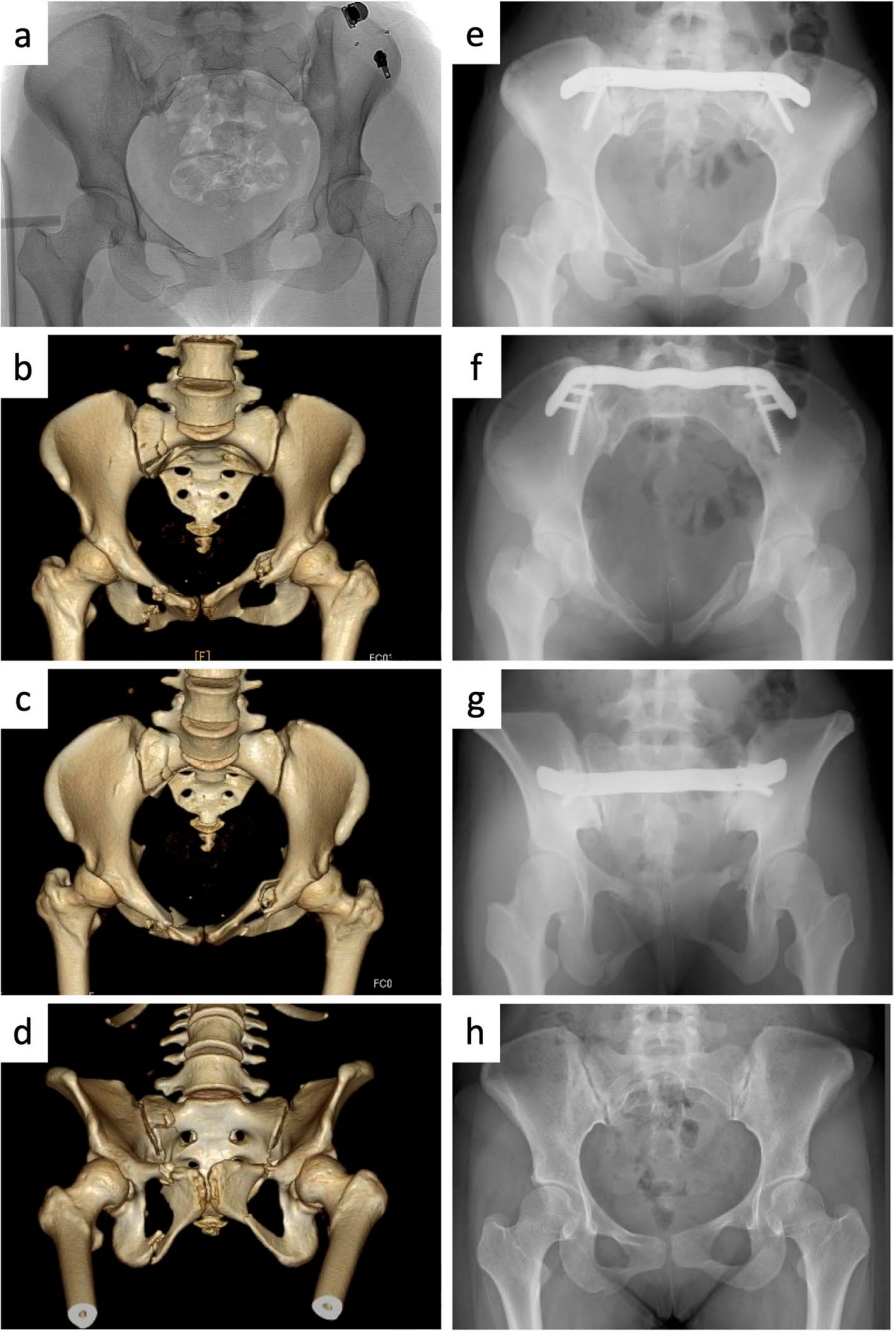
Case example. a Plain AP radiograph at the emergency room. b 3D reconstruction of the CT scan (AP view). c 3D reconstruction of the CT scan (inlet view). d 3D reconstruction of the CT scan (outlet view). e Postoperative radiograph (AP view). f Postoperative radiograph (inlet view). g Postoperative radiograph (outlet view). h Plain AP radiograph at 26 months postoperatively (after implant removal)
